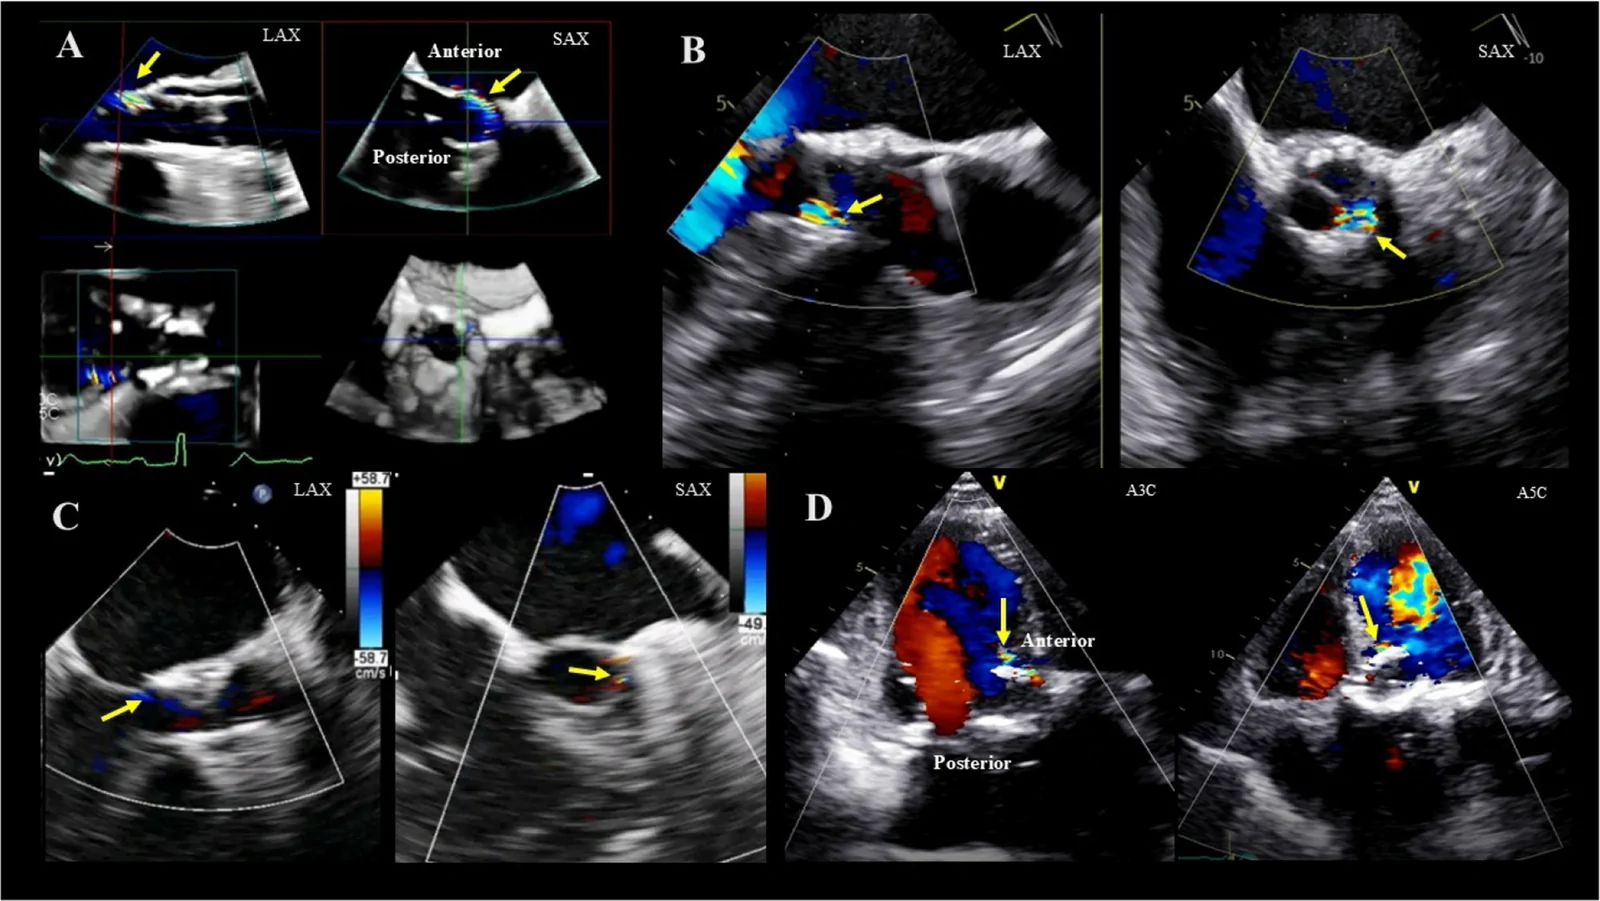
Paravalvular and transvalvular aortic regurgitation (AR) on echocardiography. A A posteriorly located paravalvular AR jet is best visualized in the mid-esophageal long-axis (LAX) view on transesophageal echocardiography (TEE) images acquired using the standard adult-TEE probe. Based on the short-axis (SAX) view in 3D TEE, the severity of the paravalvular AR is graded as moderate, involving 20% of its circumferential extent. B Transvalvular AR jet confirmed on the corresponding short-axis view, using biplane imaging in the mid-esophageal LAX view on mini-TEE. C Micro-TEE detected mild paravalvular leak (PVL) in the LAX view, confirmed on the SAX views, at 2 o’clock. D An anteriorly located paravalvular AR is best visualized on the transthoracic echocardiography, in the apical three- or five-chamber view. Due to the acoustic shadowing of the prosthetic valve, it is important to tilt the ultrasound probe in multiple directions (upward or sideways) and in unconventional planes to detect and localize the paravalvular AR. Arrows indicate PVL
